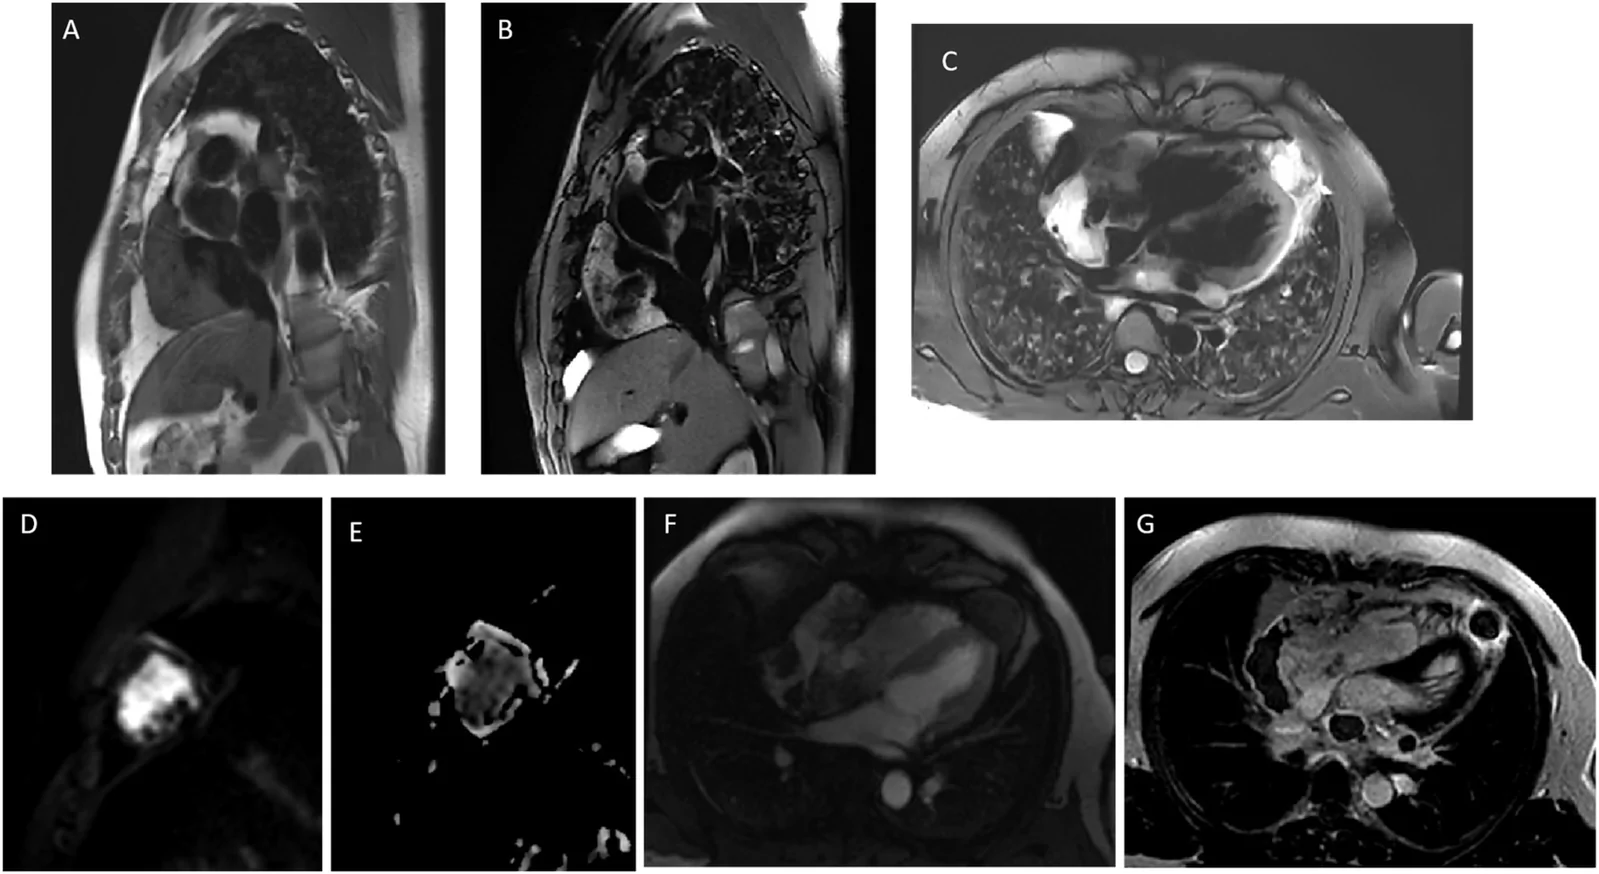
MRI (cardiac plain + function + perfusion + delayed enhancement): (A,B,C,F,G) show multiple soft-tissue nodules/masses around both atria and apex, right atrial involvement, increased mediastinal/epicardial fat, and minimal pericardial fluid. Diffuse lung nodules/patches are visible. (D,E) indicate marked diffusion restriction of lesions on DWI.
